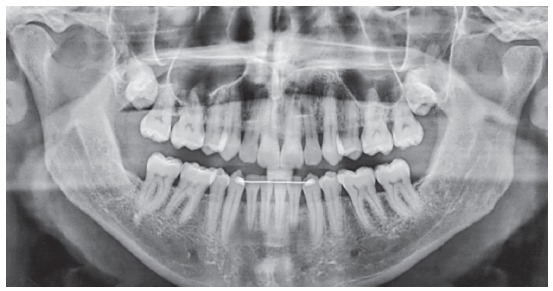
Follow-up panoramic radiograph nine years and three months after treatment completion.
